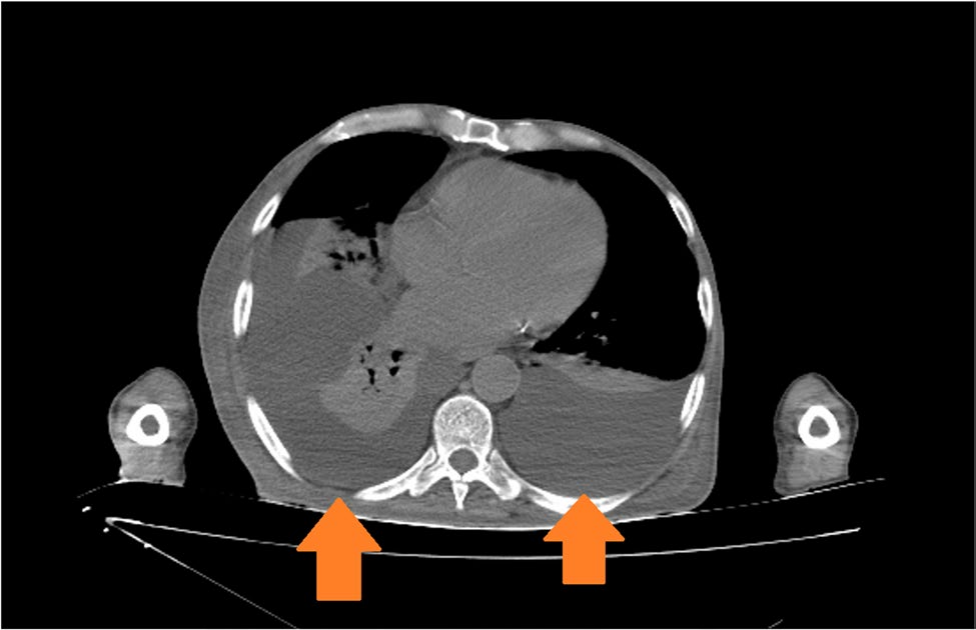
Pleural effusion image on thorax CT
